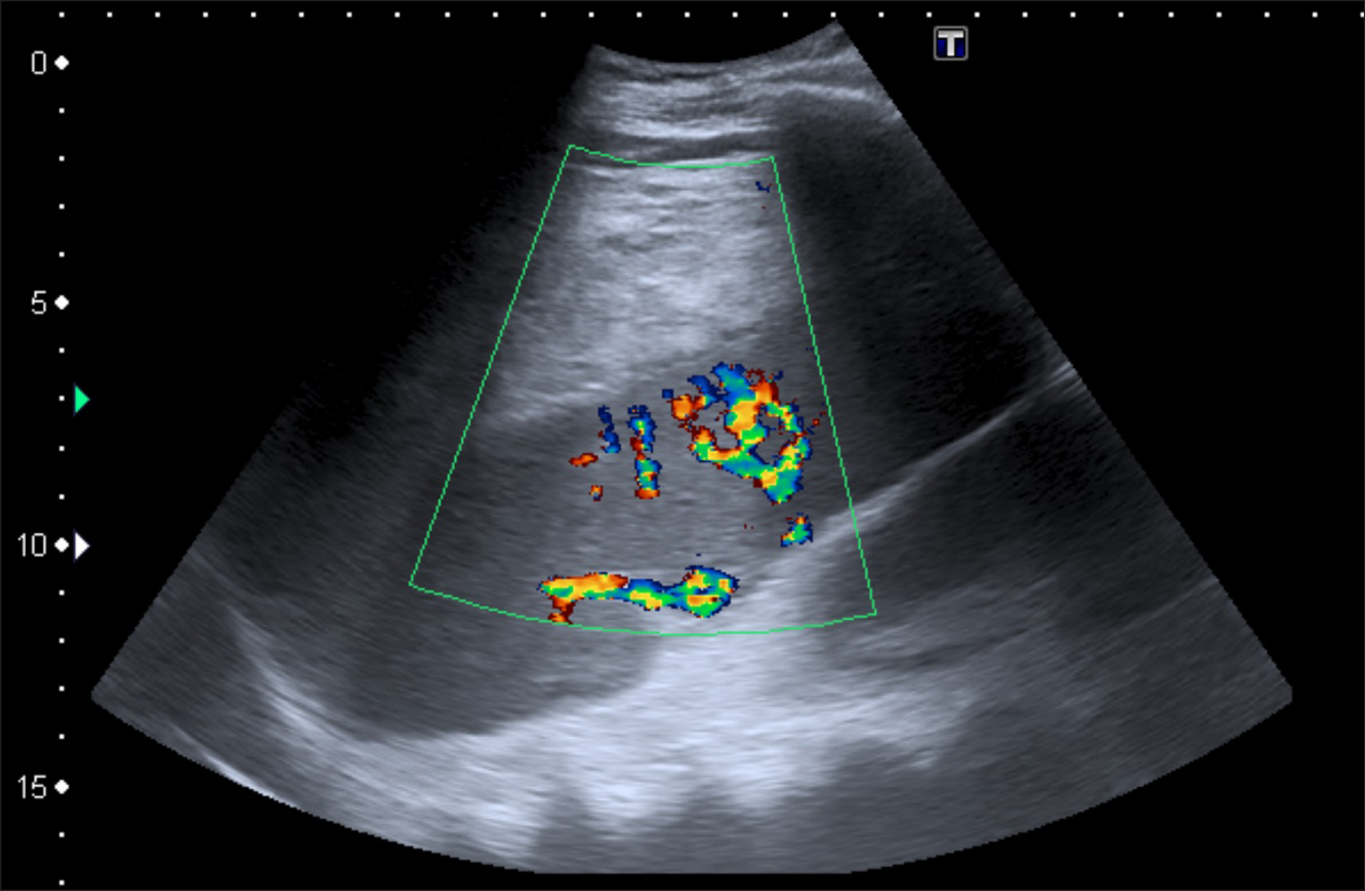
Ultrasound of the spleen with the large subcapsular hyperechoic hematoma.
